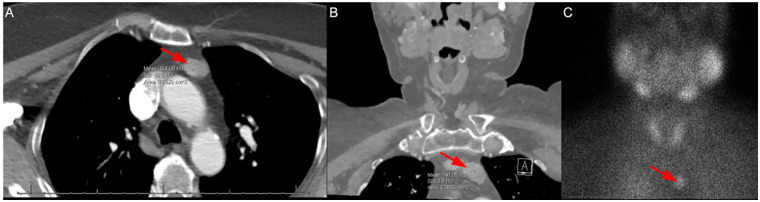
Axial (A) and coronal (B) CT images of the adenoma, with corresponding sestamibi scan (C) for the first case.
